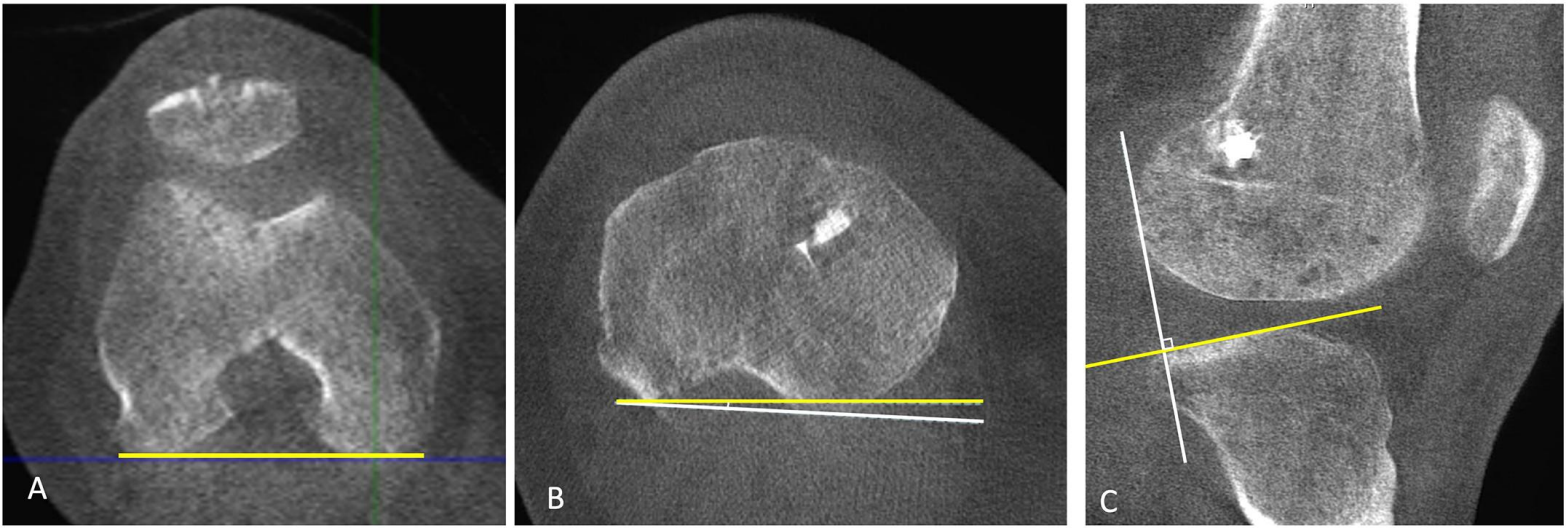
Weight-bearing CT 12 months after anatomic ACL reconstruction using interference screws for femoral and tibial fixation. Axial WBCT images (A,B) demonstrate external tibial rotation relative to the femur, consistent with restored rotational stability, and the sagittal WBCT image (C) demonstrates a negative anterior tibial translation, indicating restoration of anterior–posterior stability. The position and diameter of the tibial tunnel can be assessed on the axial image (B), while the femoral tunnel can be evaluated on the sagittal image (C).
